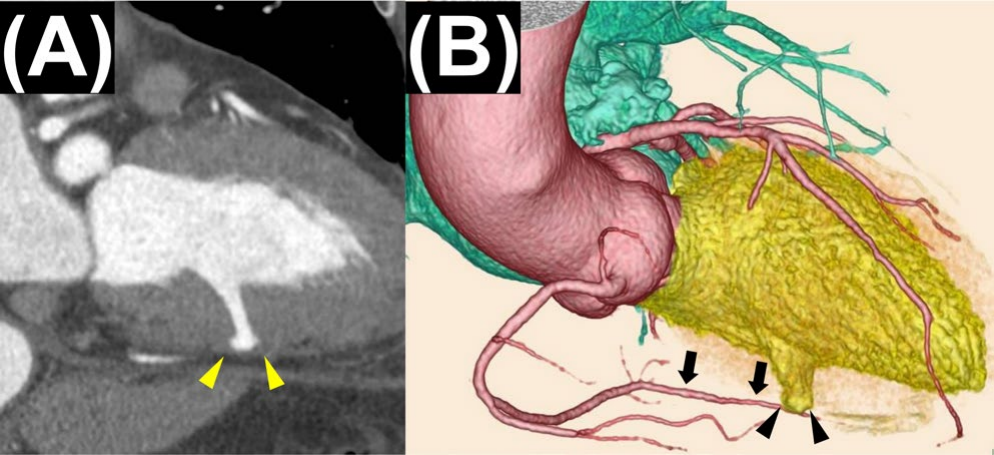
Preoperative CT images. (A) Long‐axis view demonstrating an LVA (arrowheads). (B) Three‐dimensional reconstruction showing the LVA (arrowheads) and the course of the right coronary artery to its distal portion (arrows). CT, computed tomography; LVA, left ventricular aneurysm.
